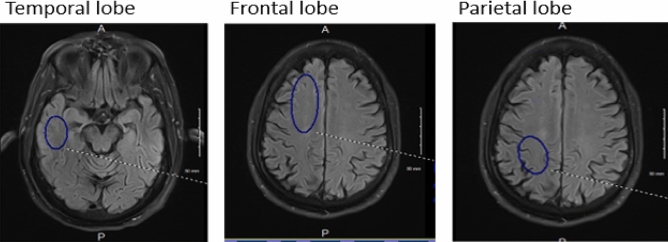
Selected brain regions chosen from the flair sequence reference scan for ROI signal averaging. The blue oval contains the region of signal average: temporal lobe 650 mm^2^; frontal lobe 1150 mm^2^; and parietal lobe 760 mm^2^. The volume, shape, and location were held constant with minimal intra and intersubject variation. Homologous contralateral determinations were also obtained. The FLAIR index scan and perfusion sequences were position locked such that the ROI was obtained from the same slice for all 7 perfusion images.
